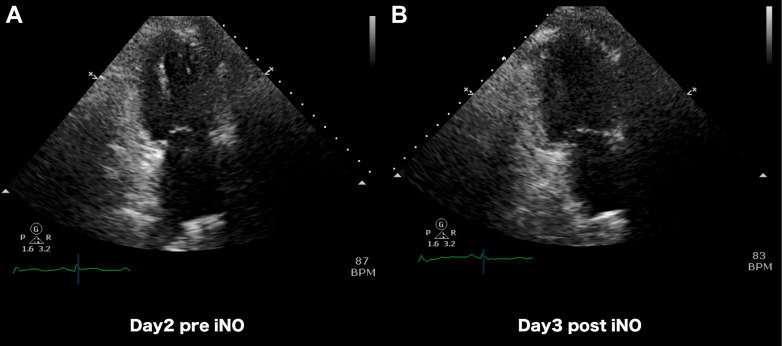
Paired Echocardiographic Images Demonstrating iNO-Induced Left Ventricular Volume Shift (Case 3) Apical 2-chamber views from case 3 at (A) day 2 baseline and (B) day 3 after inhaled nitric oxide initiation. Note the small, underfilled left ventricular (LV) cavity at baseline (A), reflecting reduced LV preload due to right ventricular (RV) dysfunction. After inhaled nitric oxide (iNO) administration (B), the LV cavity appears more filled, visualizing the mechanism whereby iNO-induced pulmonary vasodilation increased RV forward flow and LV preload. In this patient with limited LV reserve (left ventricular ejection fraction 39%), this acute volume shift resulted in LV decompensation (pulmonary capillary wedge pressure rose from 12-14 to 24 mm Hg).
